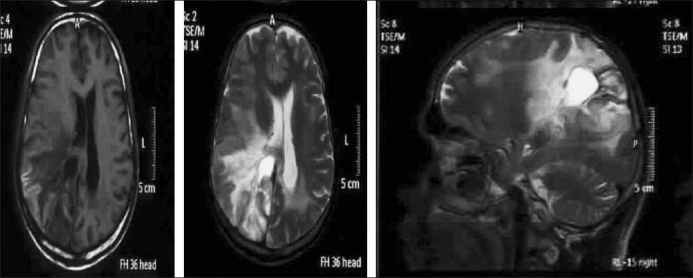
MRI of case one in the axial (T1 & T2 weighted) and sagittal views, demonstrating a mass lesion with extensive edema in the right parieto-occipital region with midline shift
